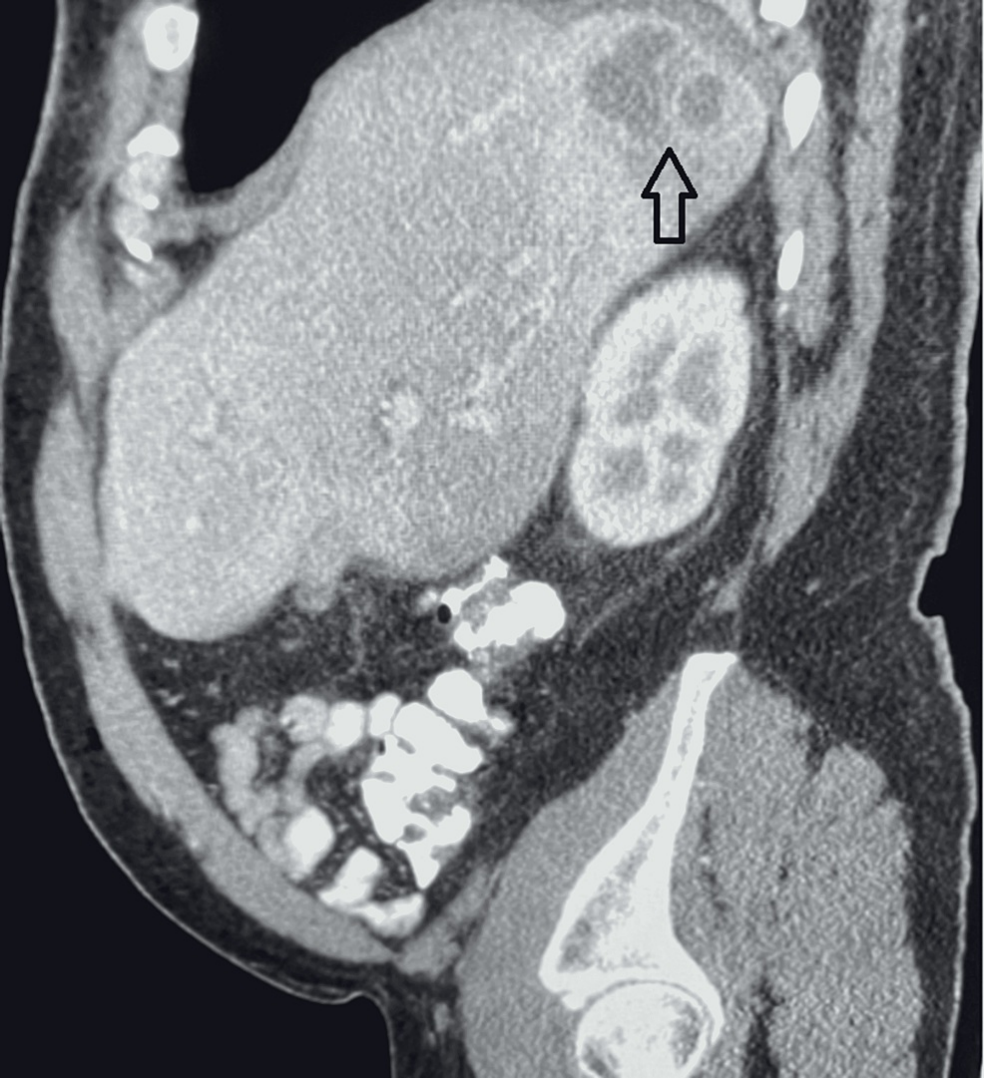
Sagittal abdominal computed tomography scan showing an abscess of the posterior segment in the right lobe of the liver (black arrow)
